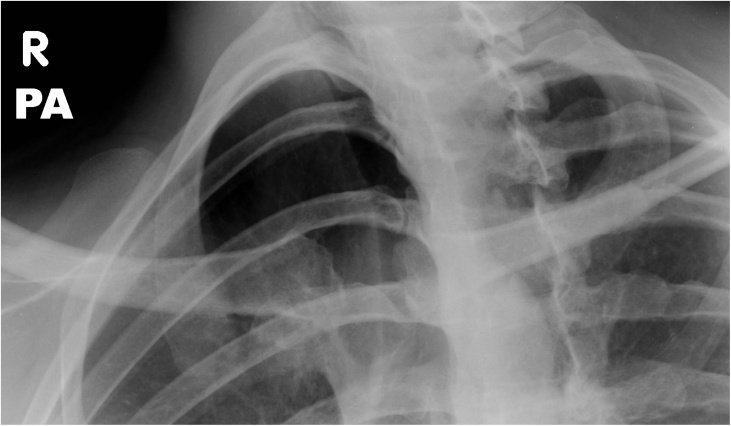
XR at 12 months follow-up. Best seen on the right oblique radiograph, the anatomic relationship of the medial aspect of the right clavicle and the right sternum is abnormal with abnormal widening but unchanged compared to the previous XR. This reflects stable posterior SCJ fracture-dislocation.
